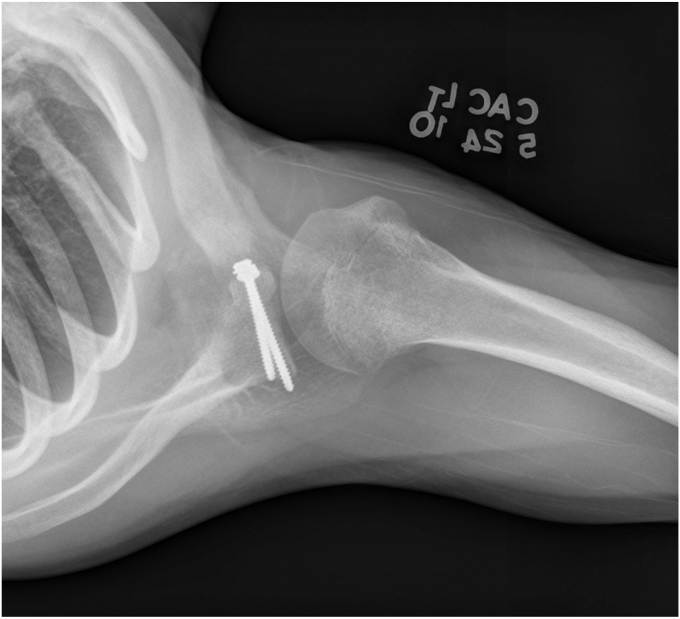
Axial X-ray from 4.5 weeks after Latarjet.
